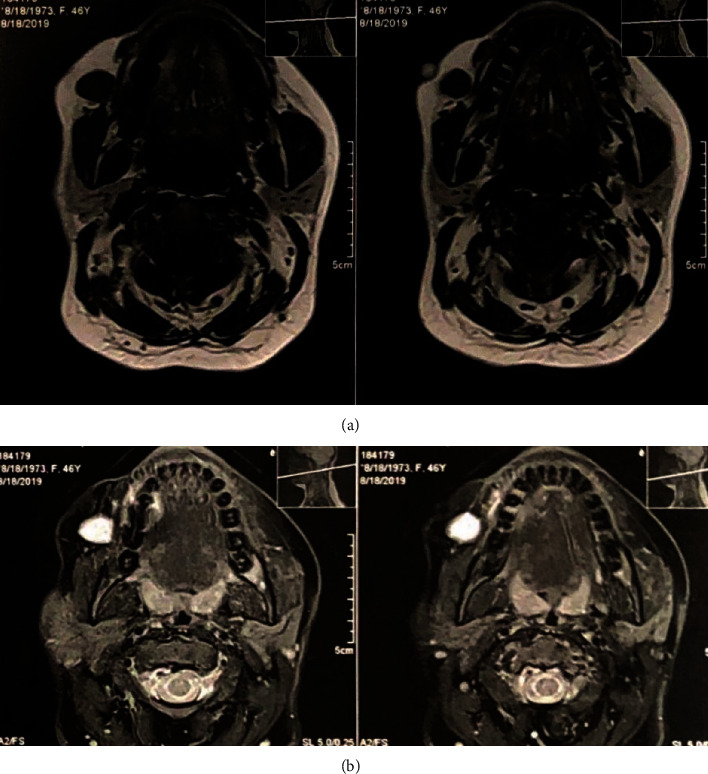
(a) Axial T1 MRI shows a low signal homogenous mass in the right lying over the buccinator muscle and under the skin. (b) Axial T2 MRI shows the lesion as high signal and homogenous.
